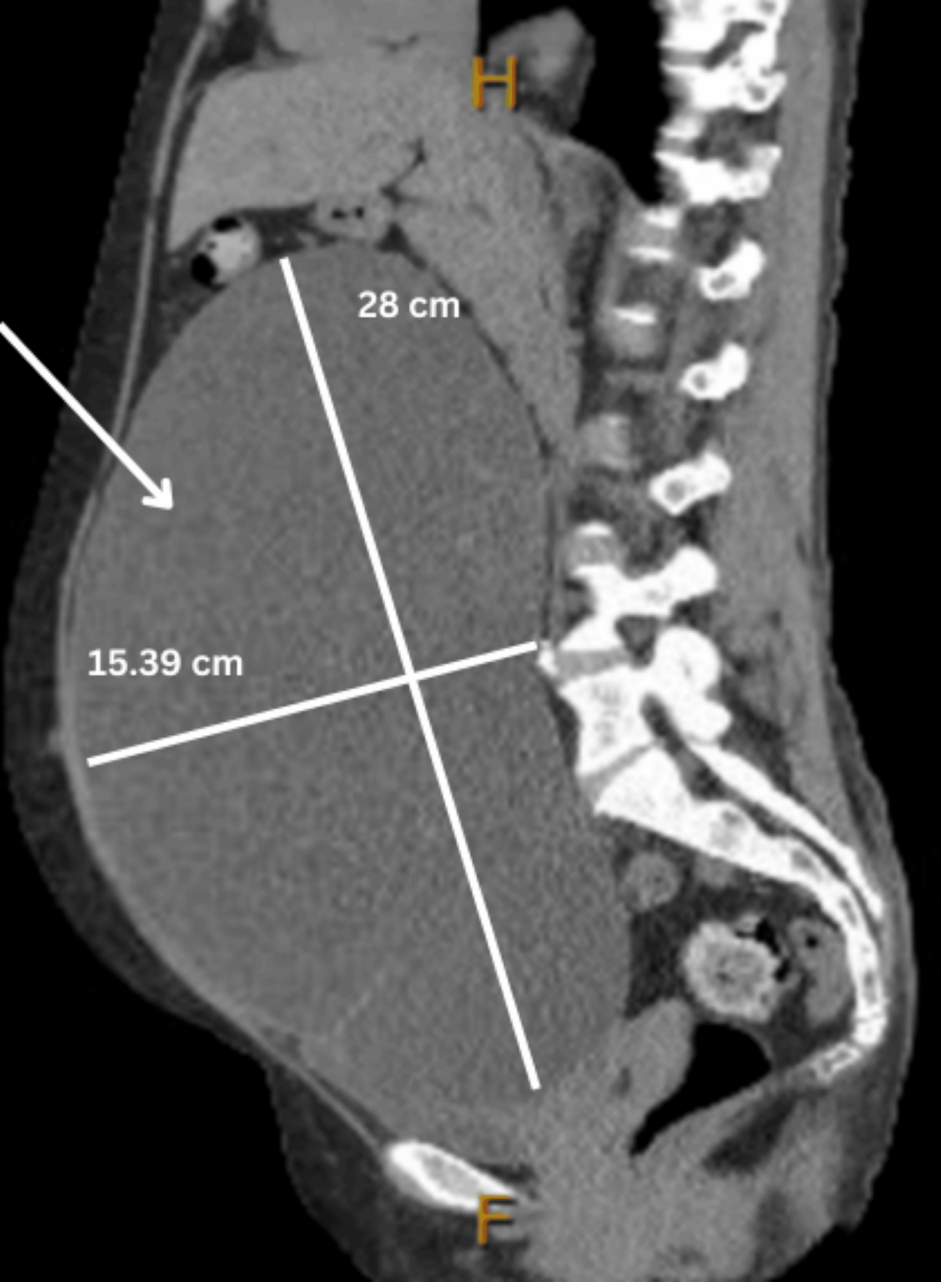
Sagittal MRI image of the right borderline ovarian tumor measuring 28 cm x 15.39 cm.
